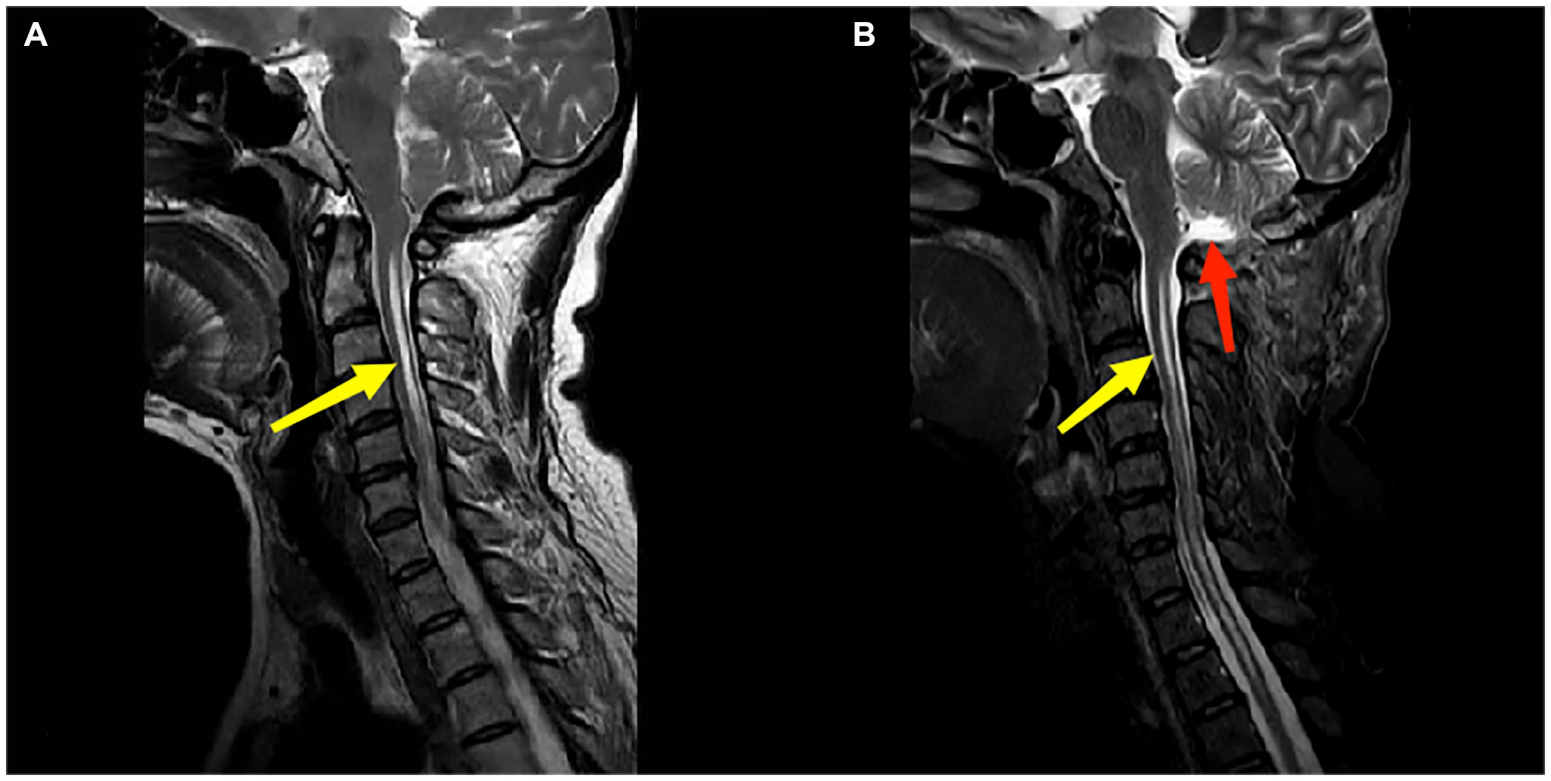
Panels (A,B) are the preoperation and postoperation MRI images of a 51-year-old female patient who underwent PFDD treatment. The red arrow in (B) indicates the postoperative recreated cistern magna. The yellow arrow indicates the patient’s syringomyelia, and there was a reduction in the diameter of syrinx postoperatively.
